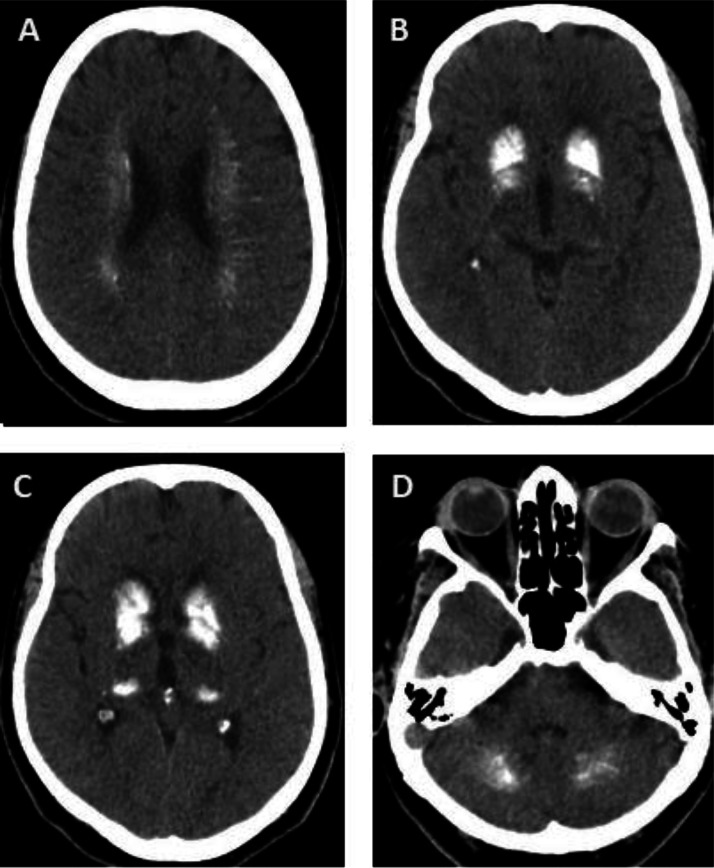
(A–D) Brain computed tomography (CT) images demonstrate extensive and bilateral calcifications in deep cerebral structures, including the corona radiata (A), basal ganglia (B and C), and dentate nuclei of the cerebellum (D). These calcifications are symmetrically distributed and predominantly affect regions involved in motor coordination and cognitive processing. The imaging findings suggest abnormal calcium deposition, which may be associated with underlying metabolic or neurological disorders and warrant thorough clinical evaluation.
